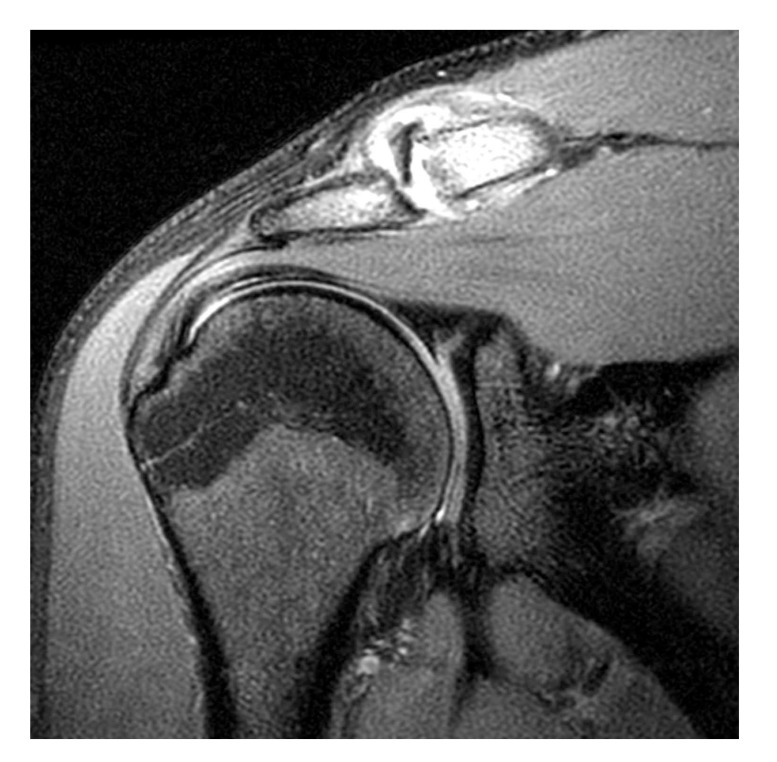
SLAP tear. Branching linear signal located posterior to the biceps anchor in a 19 year-old collegiate athlete on a coronal fat-suppressed T2-weighted image. Also note the presence of distal clavicle erosion and edema in this athlete with distal clavicular osteolysis owing to weight lifting.
